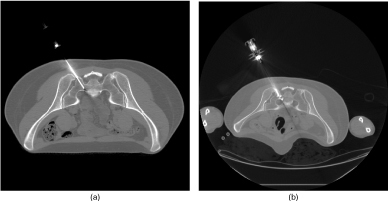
CT-guided biopsy and microwave ablation of sacral lesion. (a) Intraprocedure axial CT image (120 kVp, 90 mA, slice thickness 3 mm) of the percutaneous bone biopsy demonstrates the site of biopsy corresponded with the site of space-occupying lesion seen on MRI. Pathology confirmed intraosseous hibernoma in this location. (b) Intraprocedure axial CT image (120 kVp, 90  mA, slice thickness 3 mm) demonstrates the site of microwave ablation and cementoplasty within the same location as the probable hibernoma.
